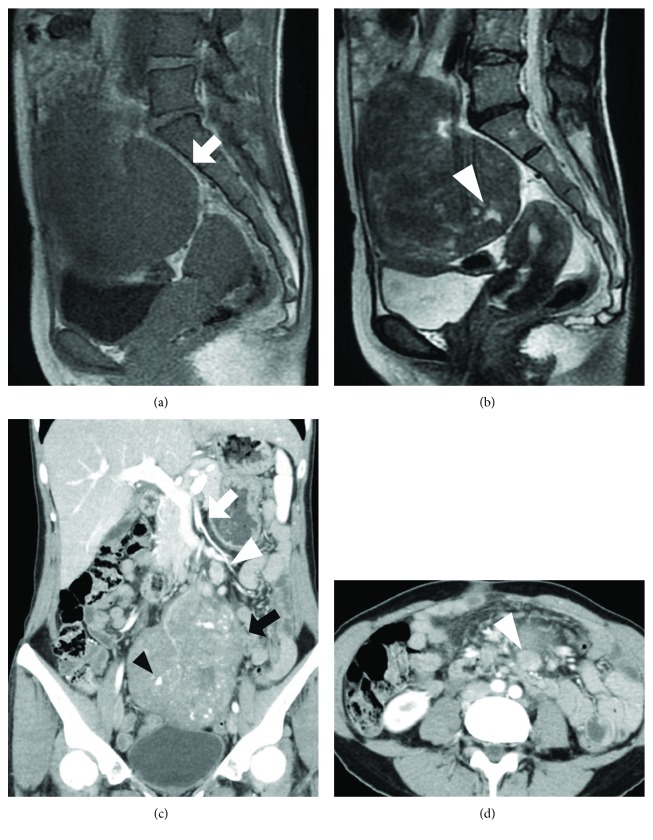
Abdominal MRI and abdominal contrast-enhanced CT. Abdominal MRI shows a homogeneous and well-demarcated 74 × 98 × 122 mm mass at the cranial part of the uterus (arrow), with a low signal intensity on T1-weighted image (a) and mostly low signal intensity and partially high intensity on T2-weighted image (b) (arrowhead). Abdominal contrast-enhanced CT shows a well-demarcated oval mass with a smooth margin and enhancement in the pelvis (black arrow). The enhanced arteries in the tumor are detected (black arrowhead). The tumor is supplied by the superior mesenteric artery (white arrow), and the surrounding lymph nodes are enlarged (white arrowhead) (c, d).
